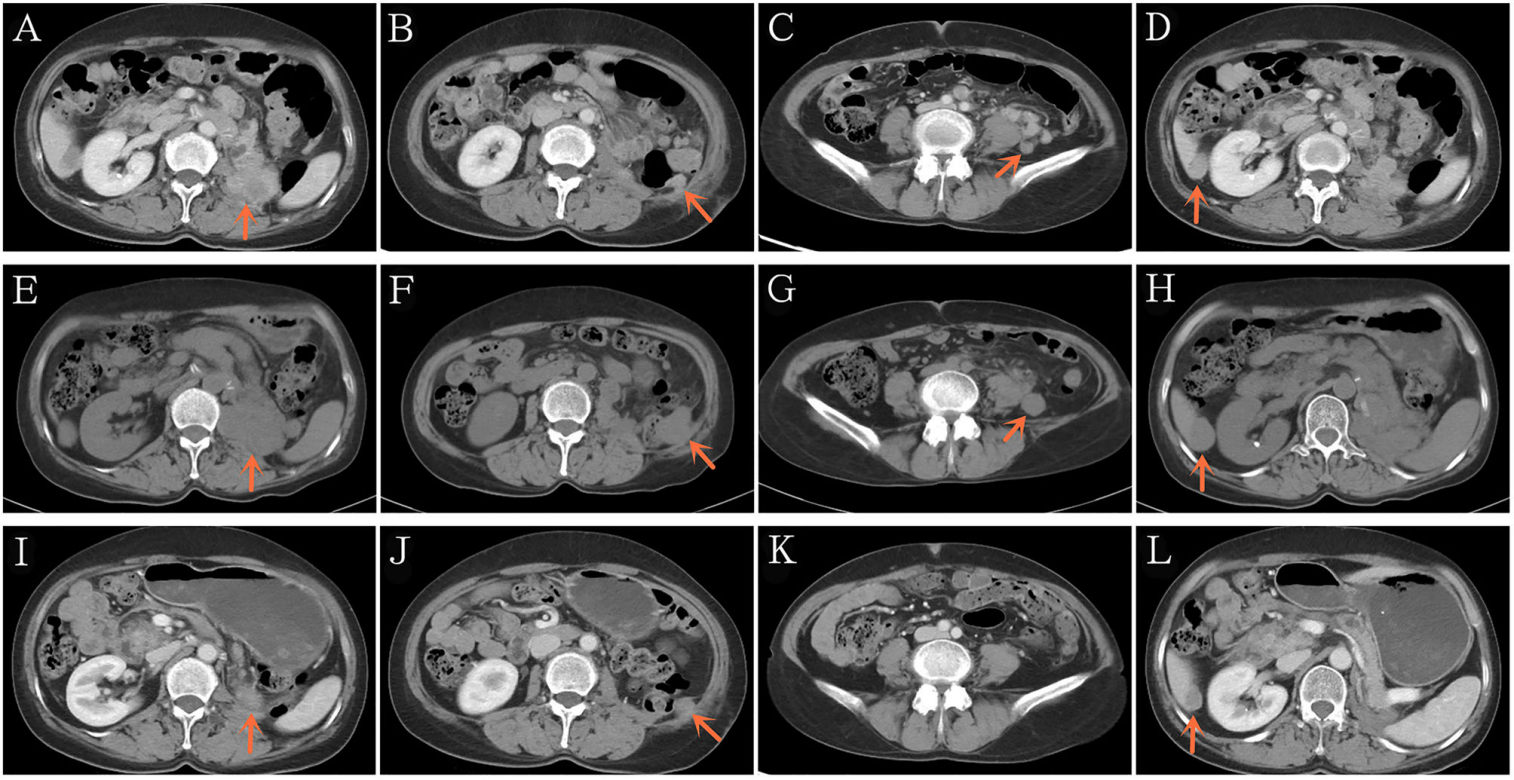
Representative computed tomography showing recurrence after 3 months of post-operative adjuvant anti-PD-1 therapy (A–D). Disease continued to progress after 2 additional months of standard-dose axitinib plus anti-PD-1 therapy (E–H). Significant reduction in the tumor burden after 2 additional months of low-dose axitinib plus anti-PD-1 therapy (I–L). PD-1/PD-L1, Programmed death-1/programmed death ligand-1.
